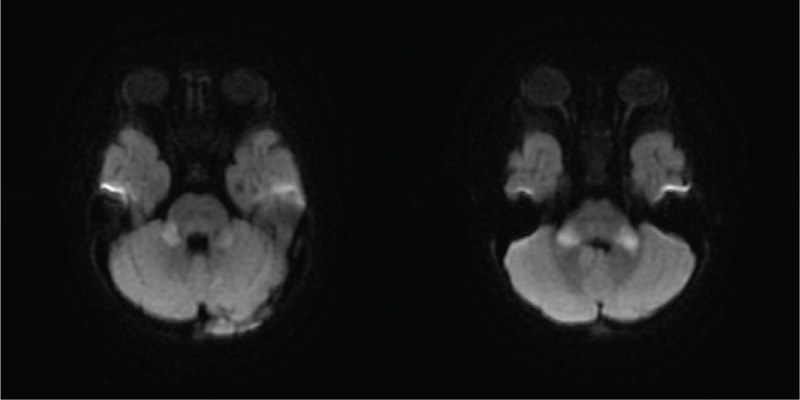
Brain magnetic resonance imaging (MRI) revealed abnormal symmetric hyperintensity in the bilateral middle cerebellar peduncles on diffusion-weighted imaging (DWI).
